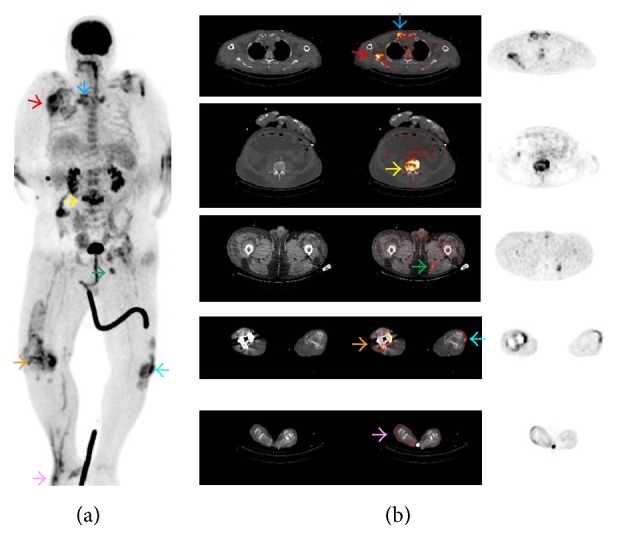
(a) MIP (maximum intensity projection) of FDG PET showing multiple areas of hypermetabolic activity corresponding to disseminated foci of infection and (b) transaxial views of CT, fused FDG PET-CT and FDG PET images representative of several foci of infection disseminated in joints, soft tissues, and lumbar column (arrows).
